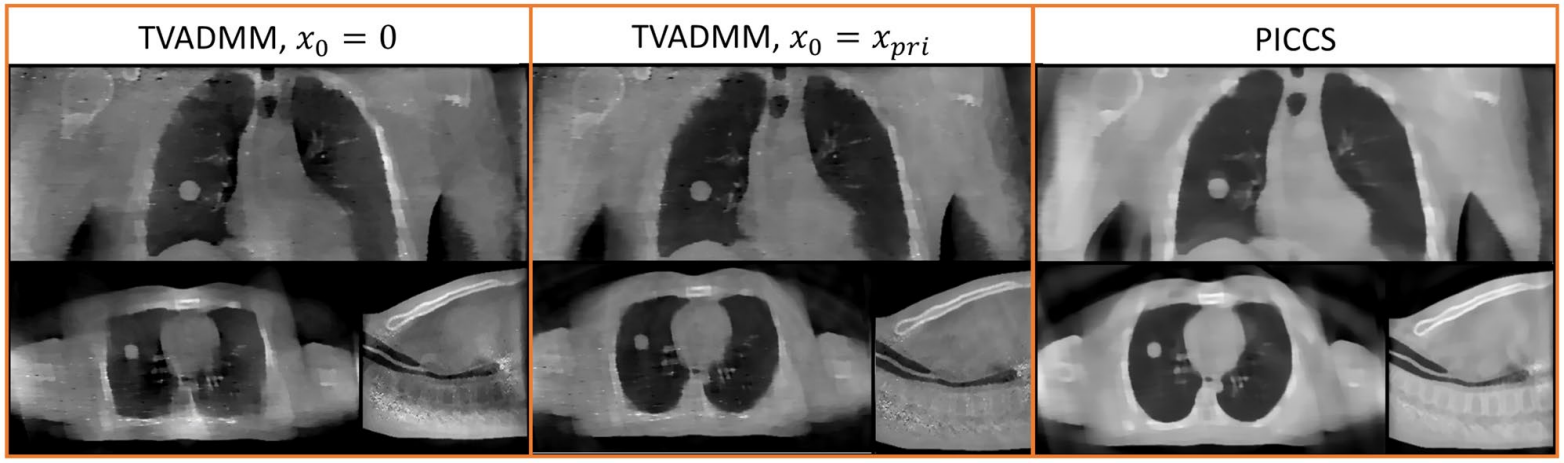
Reconstructed intra-treatment source array images. Note that $${x}_{pri}$$ is the TVADMM free-breathing CBCT image.
